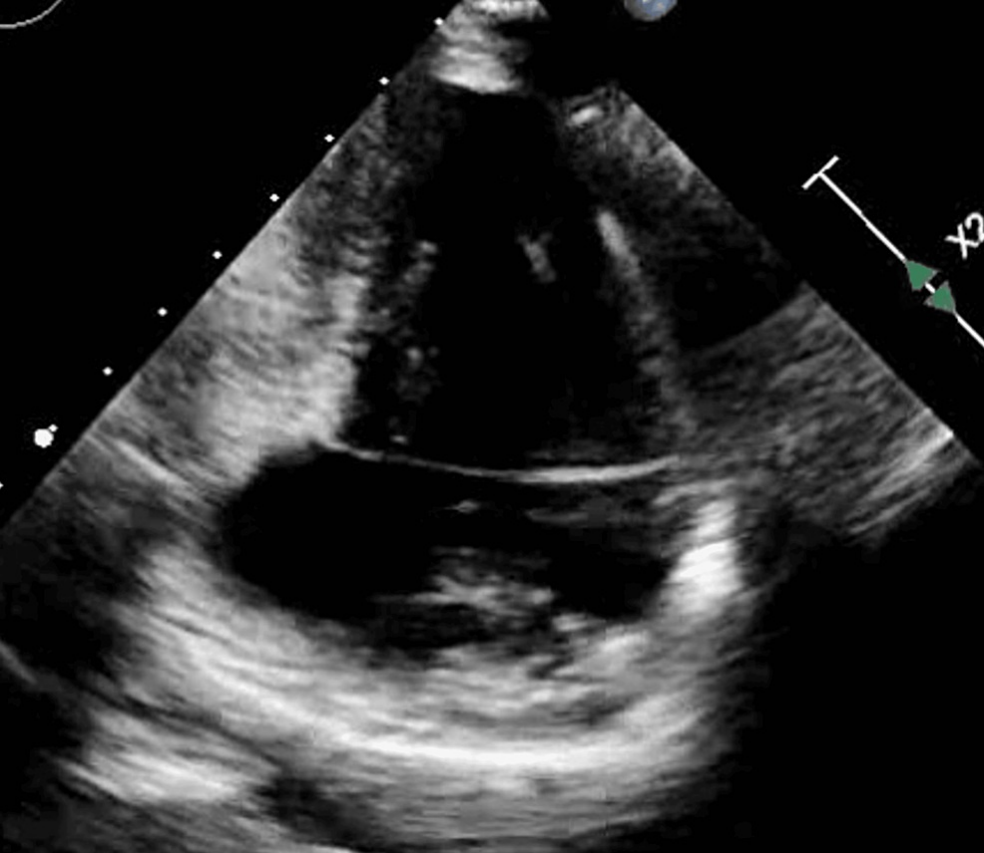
TTE TTE demonstrating basal inferior wall aneurysm in the apical two-chamber view. TTE: transthoracic echocardiogram
